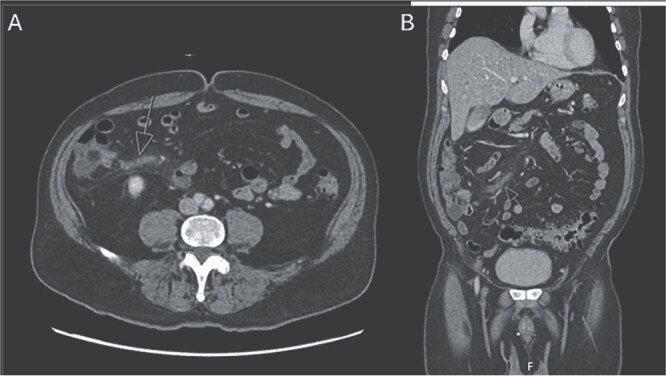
CTA abdomen and pelvis venous phase. A) Axial and B) coronal series revealing filling defect within the ileocolic vein with surrounding inflammatory changes, consistent with mesenteric thrombophlebitis of the ileocolic vein.
